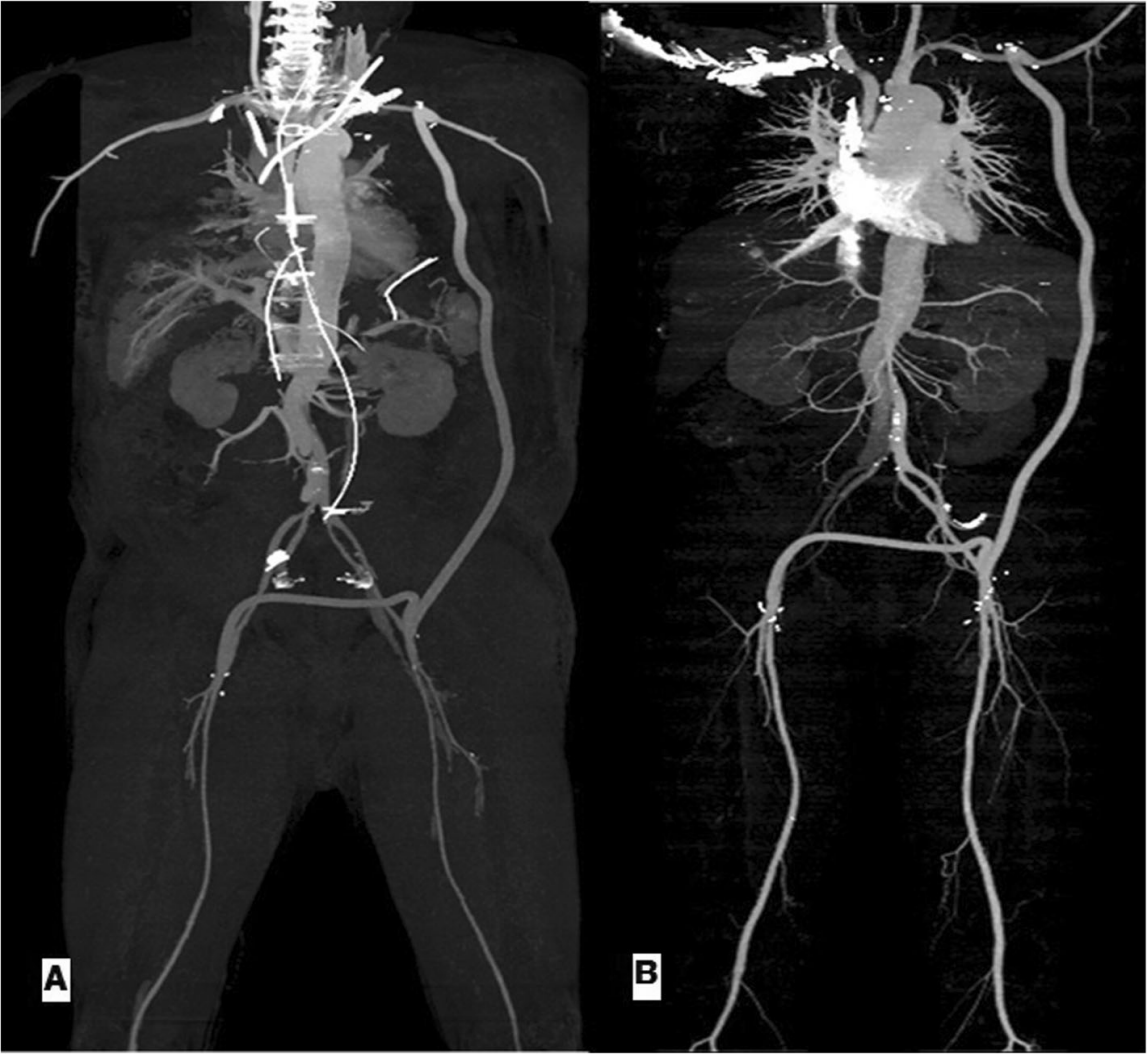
Patent left axillo-bifemoral bypass graft on follow- up aortic CTA (a: postoperative 3 days, b: postoperative 6 months). CTA, computed tomographic angiography
